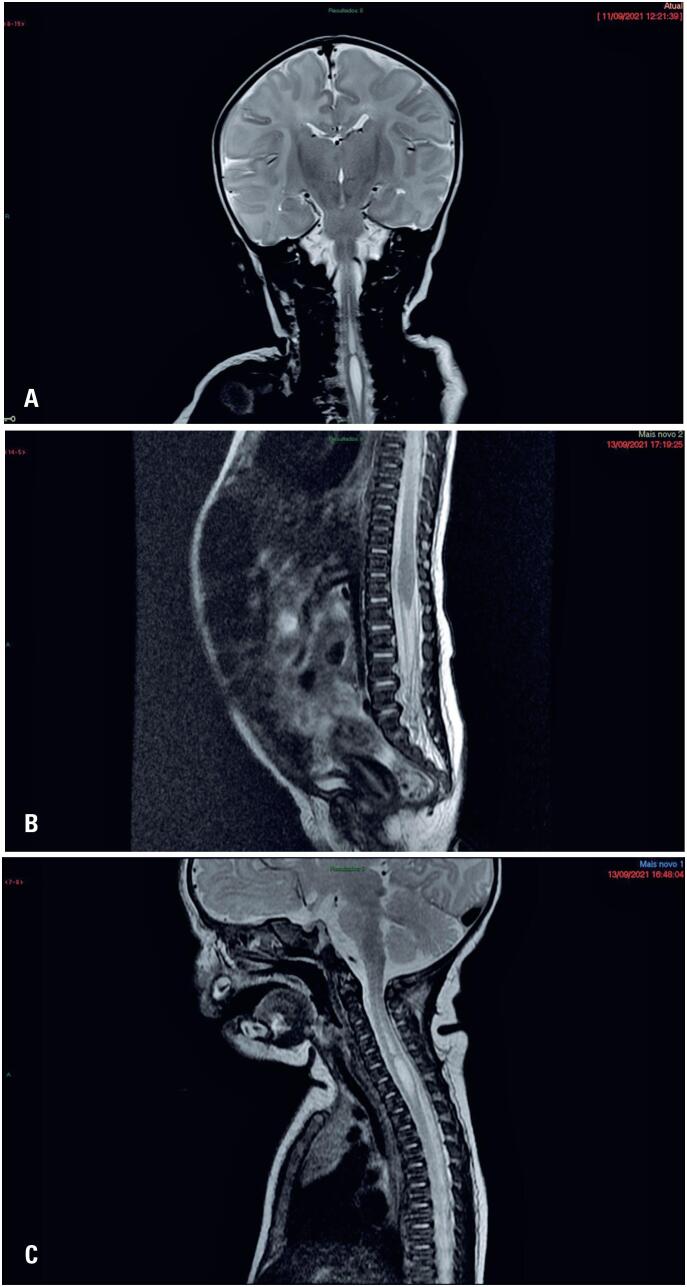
Incidental findings of cerebral and spine MRI. (A) Brain MRI - coronal T2, cervical; (B) Spine MRI - sagittal T2, lumbar thoracic; (C) Spine MRI - sagittal T2, cervical
